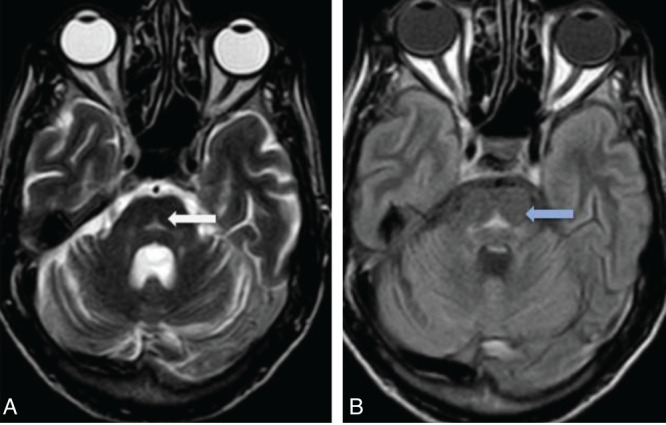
Axial T2-weighted (A) and FLAIR (B) images demonstrate a homogeneous, well-defined, triangular-shaped symmetric hyperintensity in the central pons (white arrow), without any associated mass effect. This hyperintense signal is also seen extending into the middle cerebellar peduncles (blue arrow in image B).
